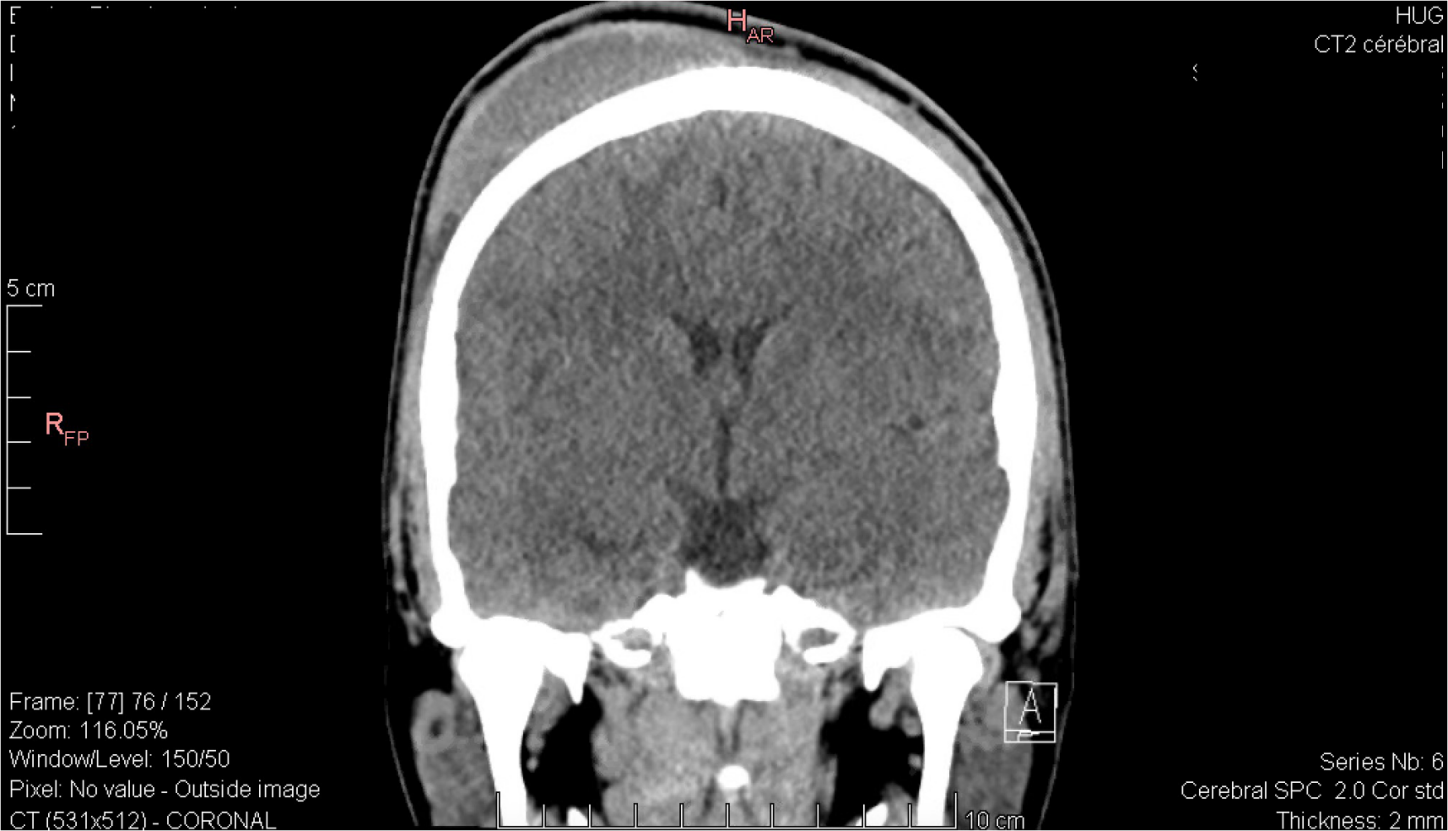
Coronal view of the head computed tomography scan revealing a large right-sided subgaleal hematoma.
